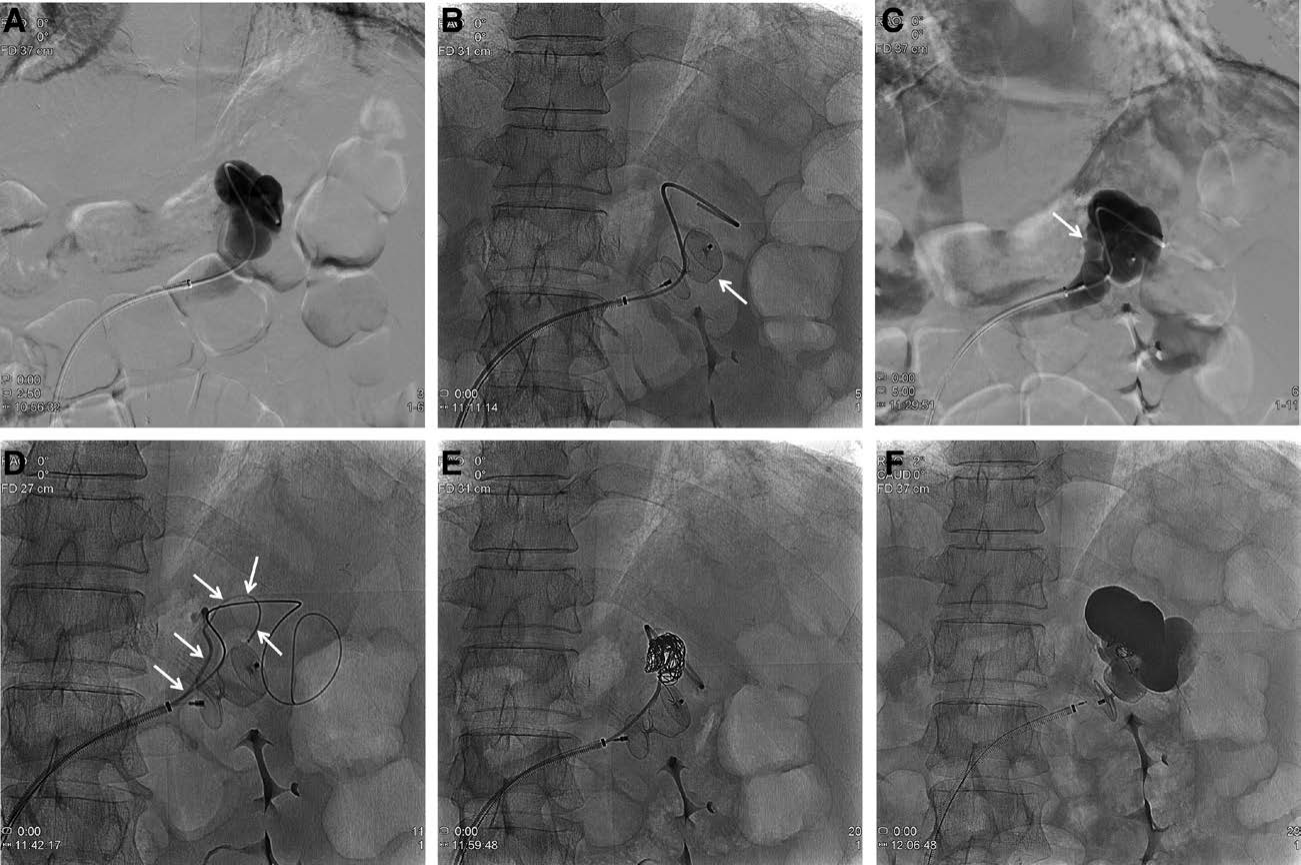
A: Venography showing a splenorenal shunt with a diameter of 16 mm. B: A 20-mm vascular plug (arrow) placed in the splenorenal shunt. C: Another splenorenal shunt (arrow) was observed on venography performed using a catheter placed distal to the vascular plug. D: This small shunt was selected using a 5-Fr. catheter and microcatheter (arrow). E, F: Coil embolization was conducted for the remaining shunt through the existing sheath, and then gelfoam was injected. CT = computed tomography.
